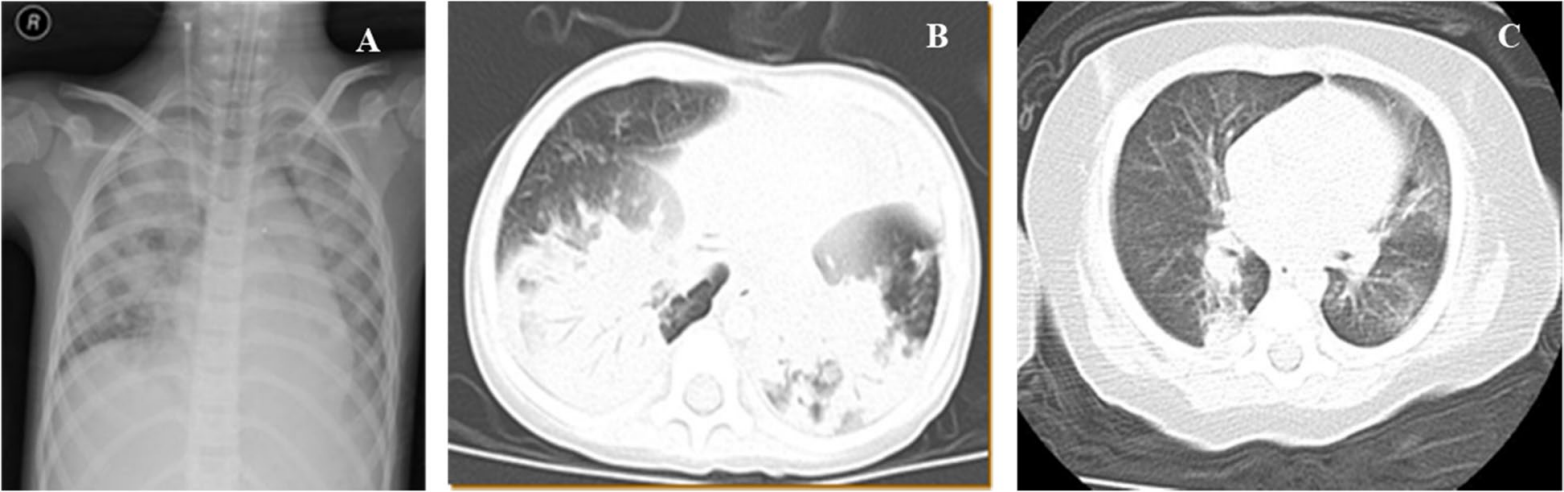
Fig. 1 Radiologic characteristic of Adv pneumonia. A X-ray showed bilateral pulmonary effusion. B Pulmonary CT showed lung consolidation. C Bronchial dilatation and transparent lung
